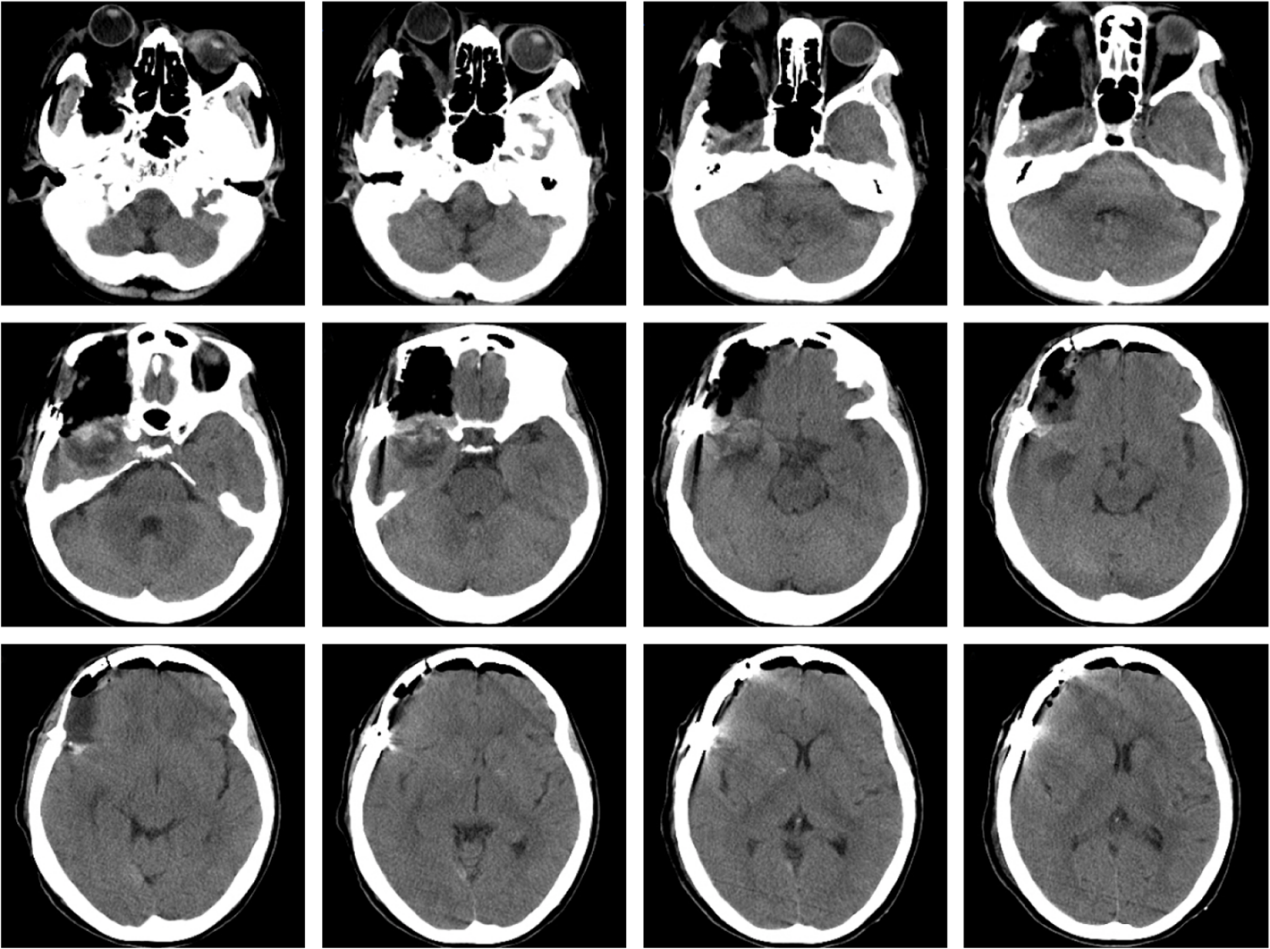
Post-operative CT scan showing the lesion was successfully resected
